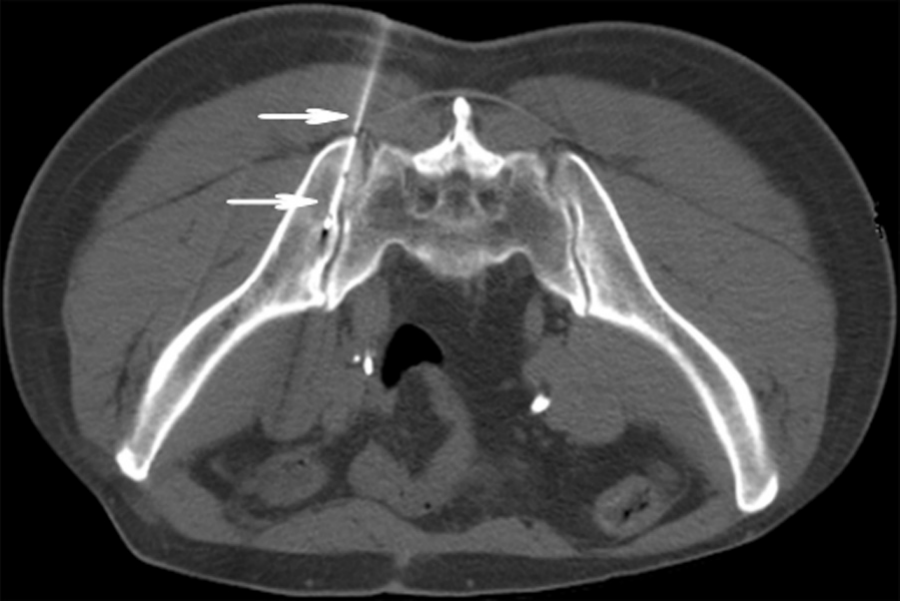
CT -guided percutaneous ^125^I seed implantation was performed according to preoperative TPS plan, a 18 G spinal needle reached the tumor (arrowhead). From deep to shallow, the particles were released.
